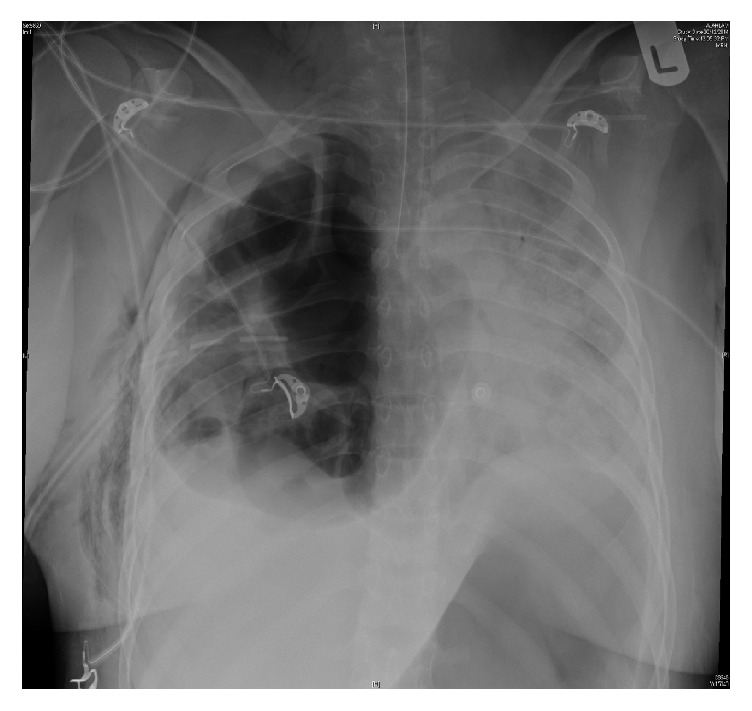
Chest X-ray showing multiple air-filled cavitary right lung lesions, right-sided pleural effusion, and progression of the air-space disease in the left lung.
